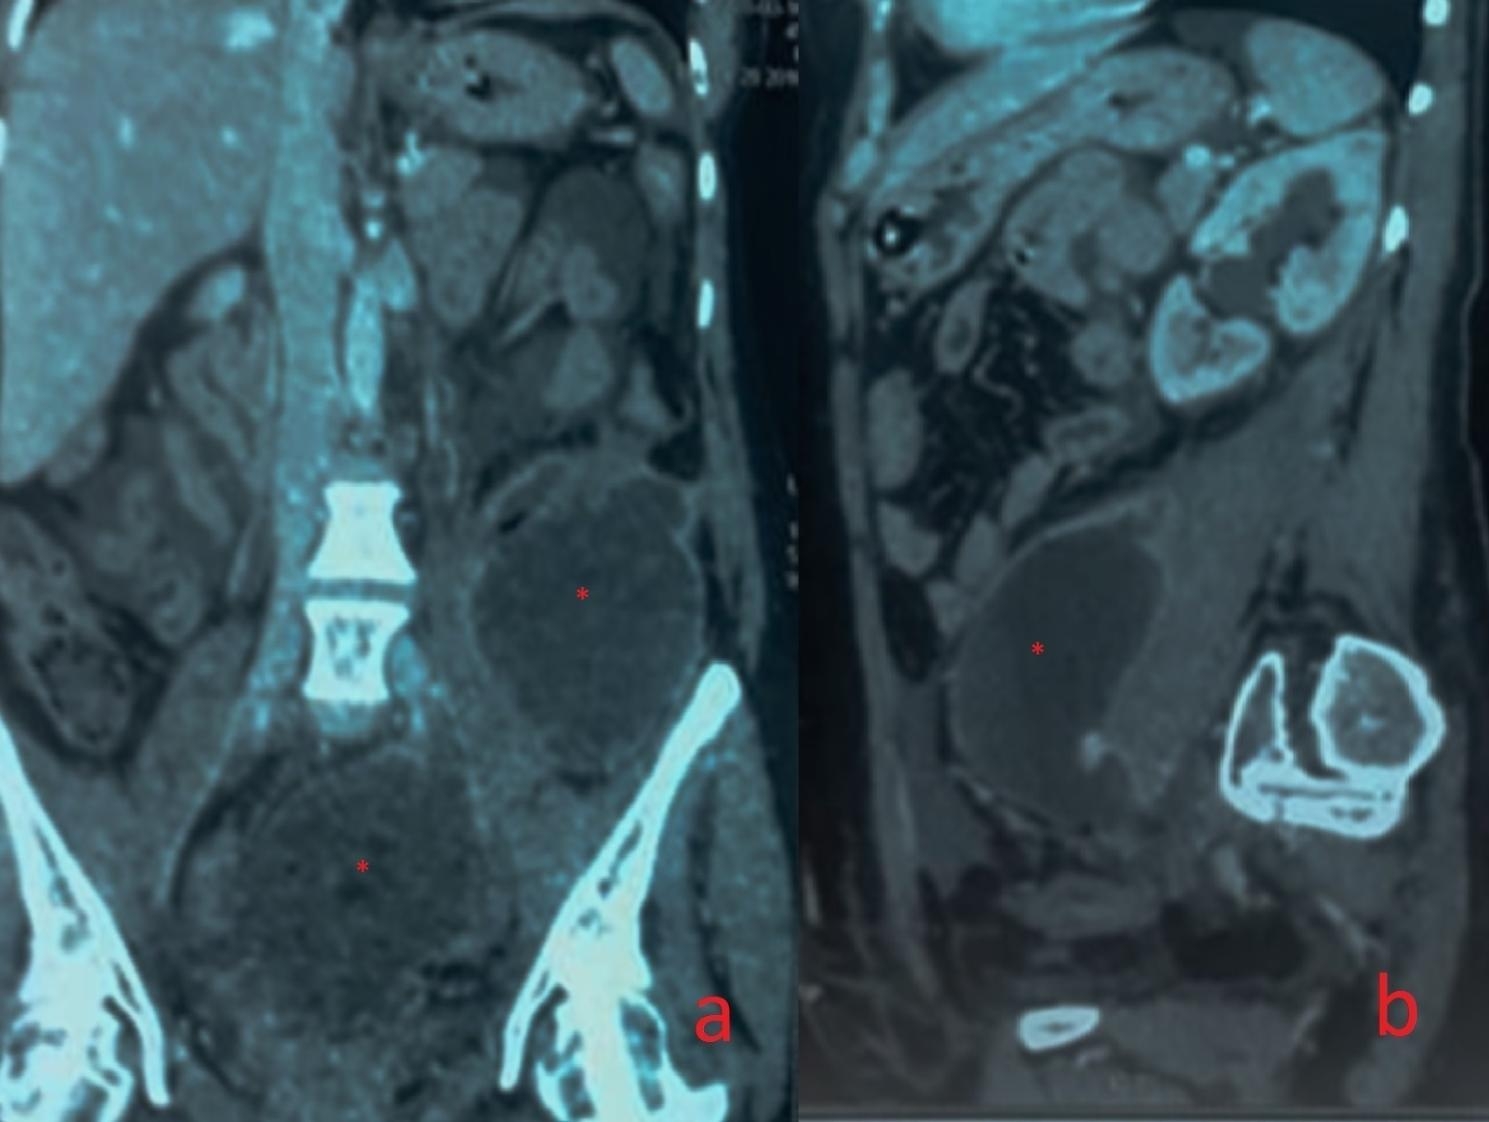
Computed tomography showing a left-retroperitoneal and pelvic urinoma (Asterisk) secondary to right iatrogenic ureteral injury (a: Frontal plane; b: Sagittal plane) after abdominal hysterectomy
